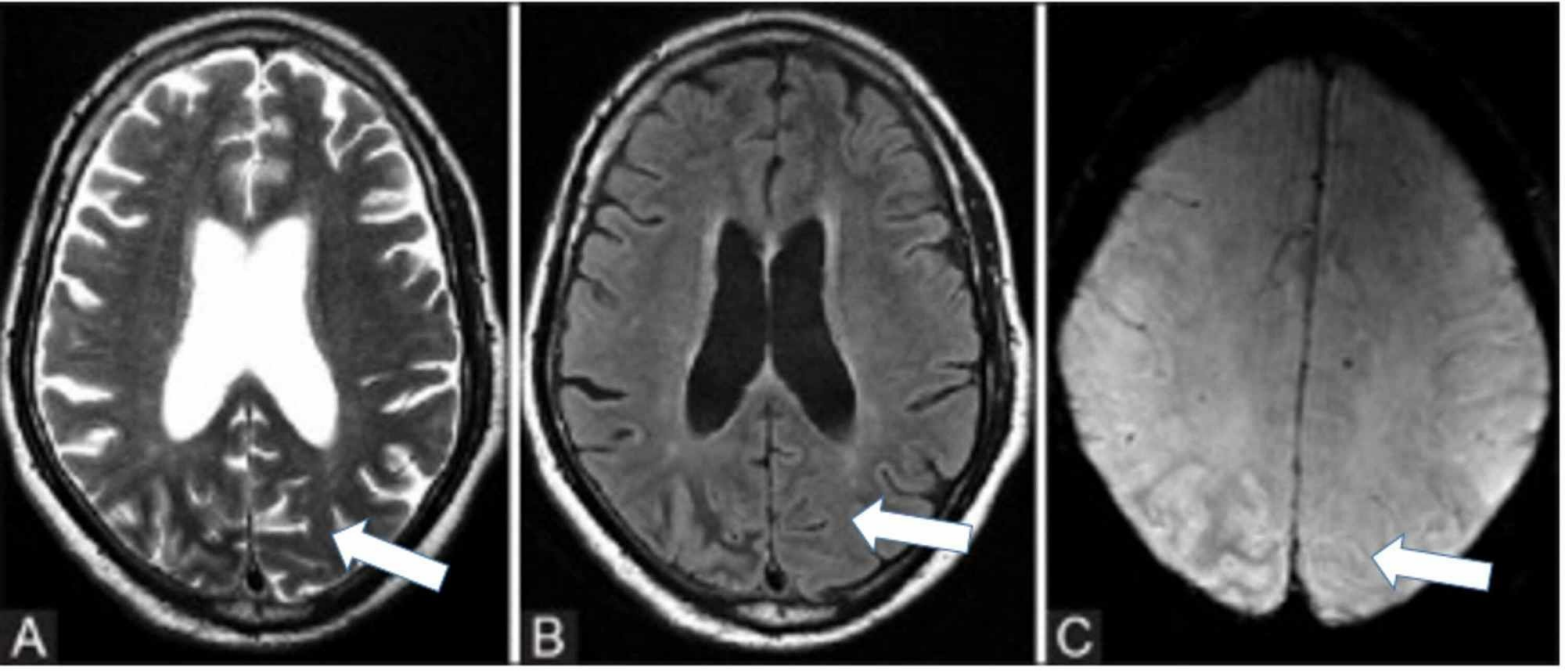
Axial T2W (A), FLAIR (B), and SWAN (C) images of the brain show cortical hyper-intensity on T2W, FLAIR, and SWAN images involving the right parieto-occipital region with adjacent subcortical white matter hypo-intensity in a patient with NKH-related occipital lobe seizure Courtesy: Dr. Shivaprakash B Hiremath T2W: T2 weighted; FLAIR: fluid-attenuated inversion recovery; SWAN: star-weighted angiography radiology; NKH: non-ketotic hyperglycemia
